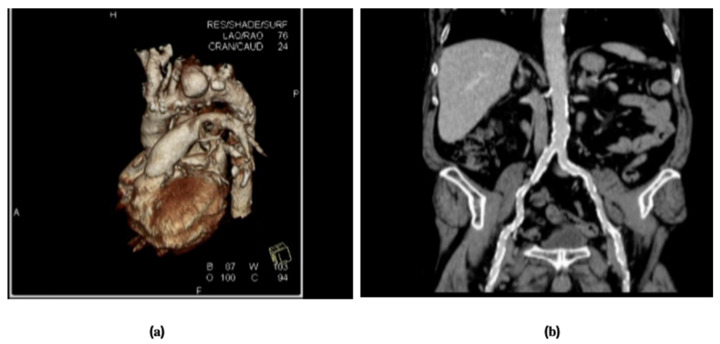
Pre-operative CT-scan: (a) 3D reconstruction of distal arch pseudo aneurysms due to penetrating aortic ulcers; (b) coronal view showing severe calcific occlusive disease of infra-renal aorta and peripheral arteries.
